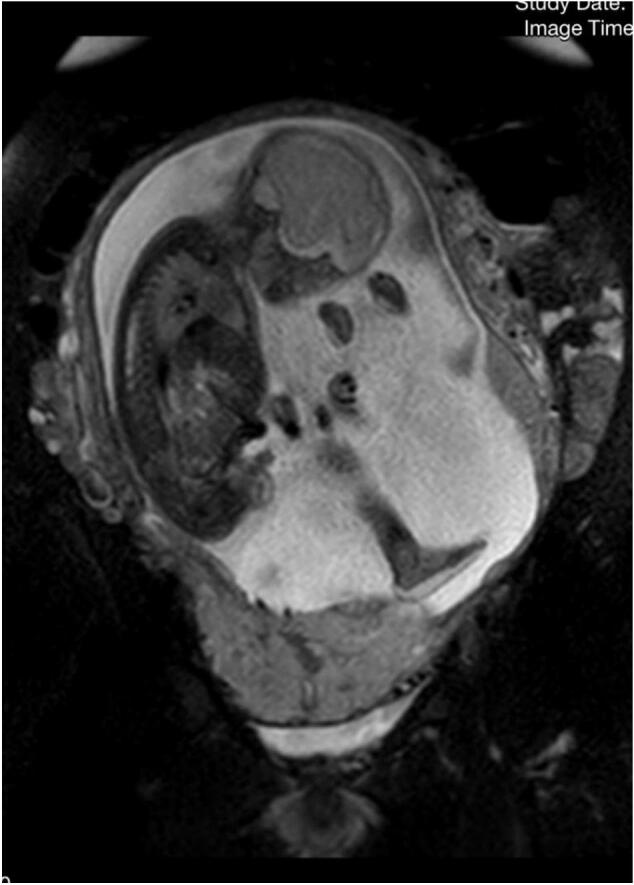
Coronal T2 weighted abdominal magnetic resonance image of invading placenta to the myometrium.
